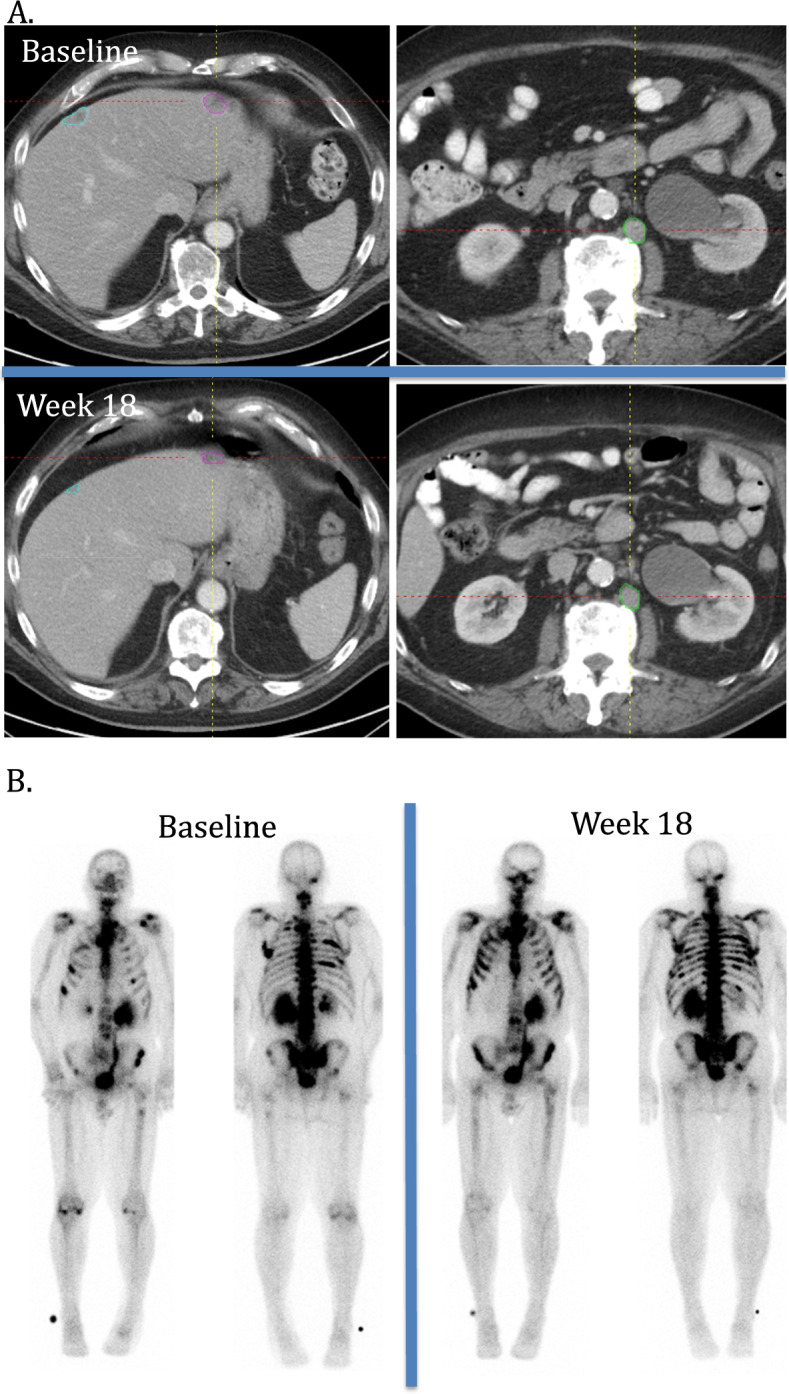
Example case: Baseline visceral disease with worsening disease by BSLA bone scan only as source of disease progression. A. Axial CT of the abdomen at baseline and week 18. Soft tissue target lesions at baseline include liver (blue and pink circles) and retroperitoneal lymph node (green circle) and were stable and slightly smaller at week 18. B. AP and PA Tc-99 bone scans were also performed at baseline and week 18. Multiple bone lesions are present at baseline, with increased and new lesions involving ribs bilaterally and right pelvis at week 18
